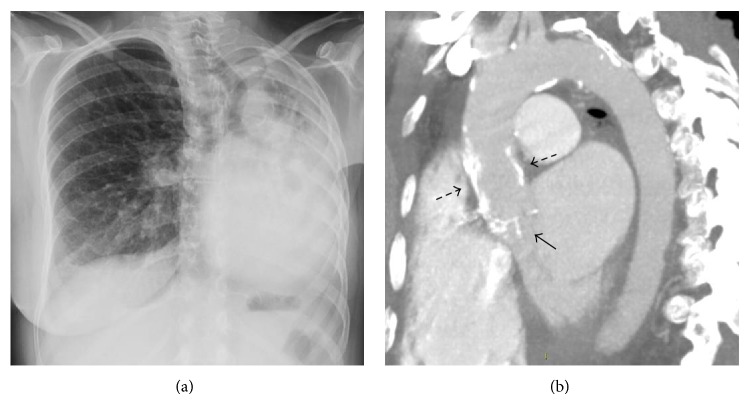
(a) Chest X-ray demonstrating left fibrothorax with ipsilateral mediastinum and trachea displacement. (b) CT scan demonstrating thickening and calcification of the aortic valvular leaflets (arrow) and diffuse calcification of the ascending aorta (dashed arrows).
